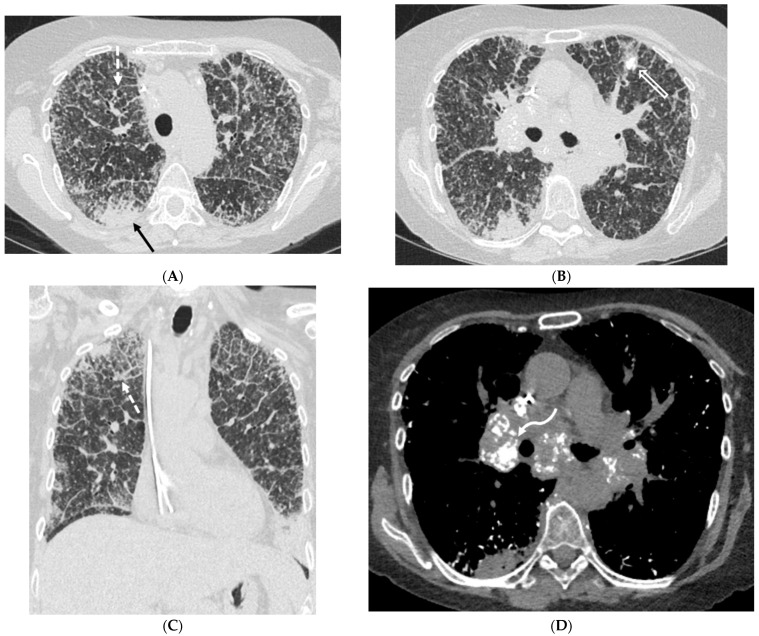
A 64-year-old female presenting with a history of amyloidosis. Axial CT images through the upper and mid thorax (A,B) and a coronal CT image (C) demonstrate diffuse nodular interlobular septal thickening (dashed white arrow) and innumerable micronodules throughout the lungs (open white arrow). Ill-defined areas of peripheral consolidation (solid black arrow) are also seen with a predilection for lower lung zones. An axial CT image displaying soft tissue windowing (D) demonstrates punctate calcifications within the lungs as well as partially calcified mediastinal and bilateral hilar lymphadenopathy (curved white arrow).
